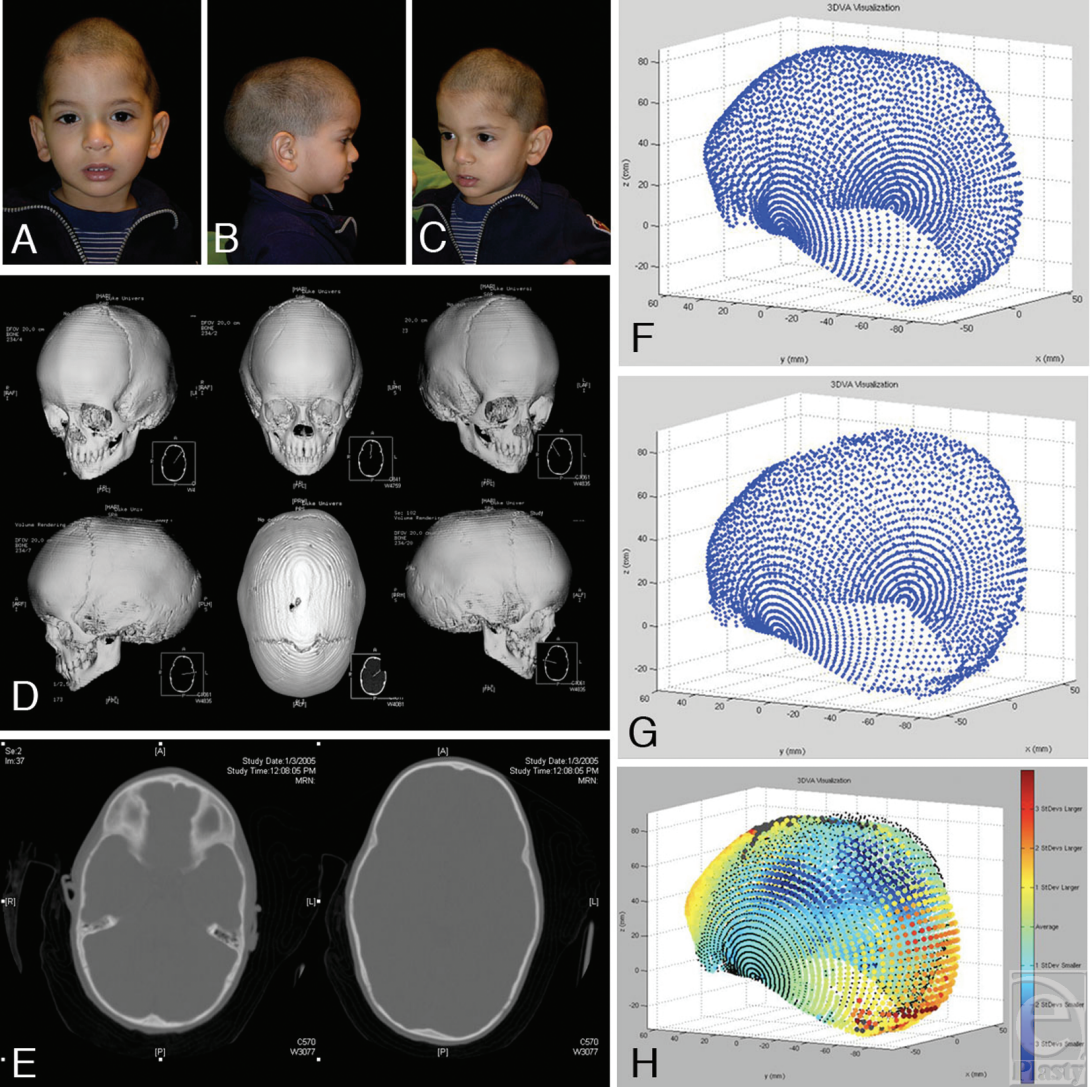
Sagittal synostosis. (A, B, and C) Nine-month-old with narrow bitemporal width, AP elongation, high anterior vertex, but no frontal bossing. (D and E) 3D CT demonstrating sagittal synostosis. The closed metopic suture perhaps contributed to this particular shape pattern, atypical for scaphocephaly. (F) 3DVA-generated point cloud for the patient's DICOM data. (G) 3DVA-averaged data for normal 9-month-old males (*N* = 4). (H) Subject's data set (colored points) compared with normative data set (black points). The color scale describes each point's distance (in number of standard deviations) from the population mean. Note anterior vertex and low occipital bossing and high occiput recession.
